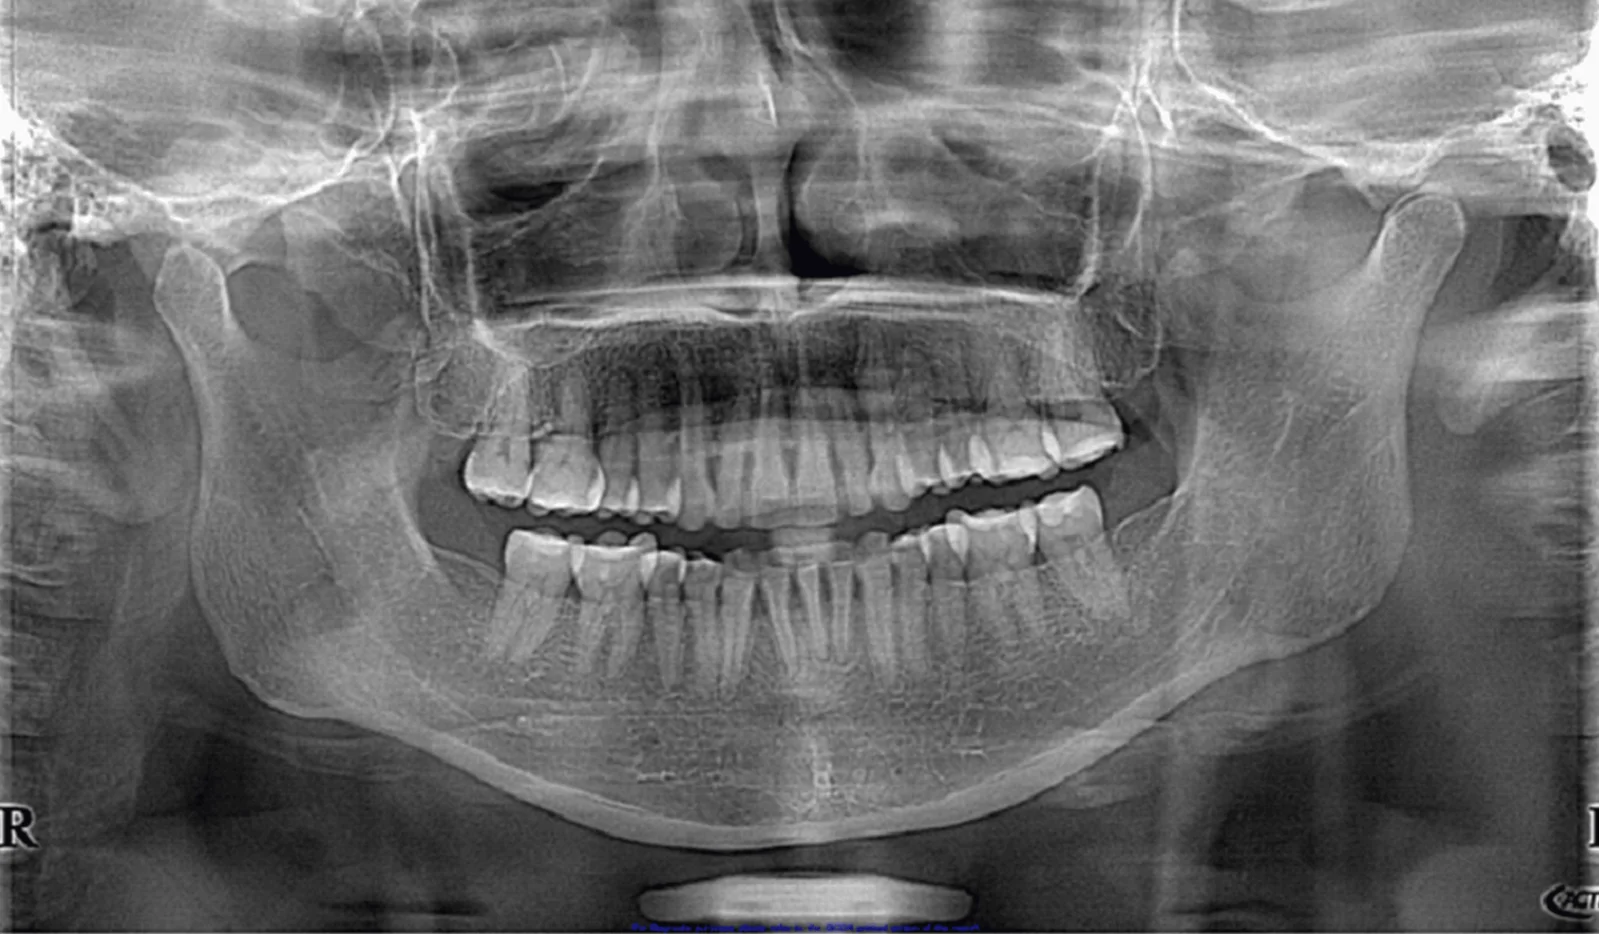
Preoperative panoramic radiograph. Preoperative panoramic radiograph obtained during the diagnostic evaluation. The image demonstrates generalized dentition with no significant periodontal bone loss or other radiographic pathology. The mandibular anterior teeth exhibit normal supporting alveolar bone levels, and the radiographic findings were used in conjunction with the clinical examination and cone-beam computed tomography for comprehensive treatment planning.
